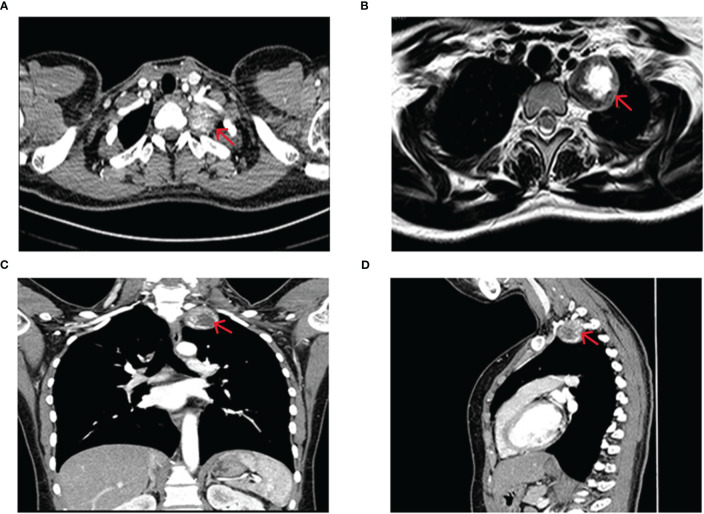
Imaging before surgery. (A) CT scan of the chest showing a 33 mm mass with clear boundaries located beside the mediastinum of the upper lobe of the left lung. Calcified nodules within the mass are slightly enhanced. Coronal (C) and sagittal (D) chest CT images reveal no abnormal changes in the adjacent thoracic vertebrae and ribs. (B) MRI of the thoracic vertebrae showing an equal-length T1, and long T2 mainly mixed signal shadows on the left side of the upper mediastinum (around the level of the T1-T2 vertebrae), low-signal shadows are observed on T2WI.
